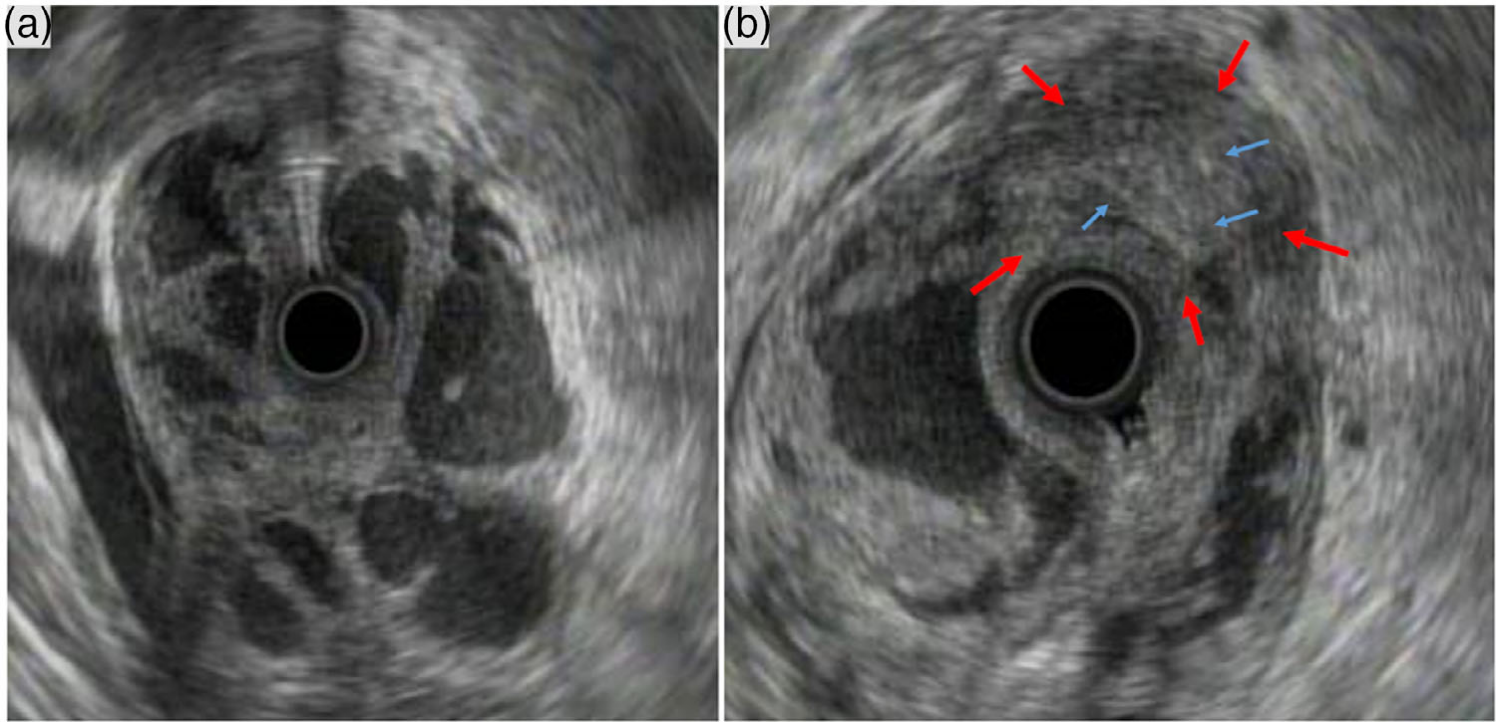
Endoscopic ultrasonography findings. Multiple submucosal cysts at the gastric body and antrum (a). At the gastric antrum, the submucosal tumor within the muscularis propria (b, red arrows) with scattered linear echoes inside (b, blue arrows) were the findings consistent with the ectopic pancreas.
